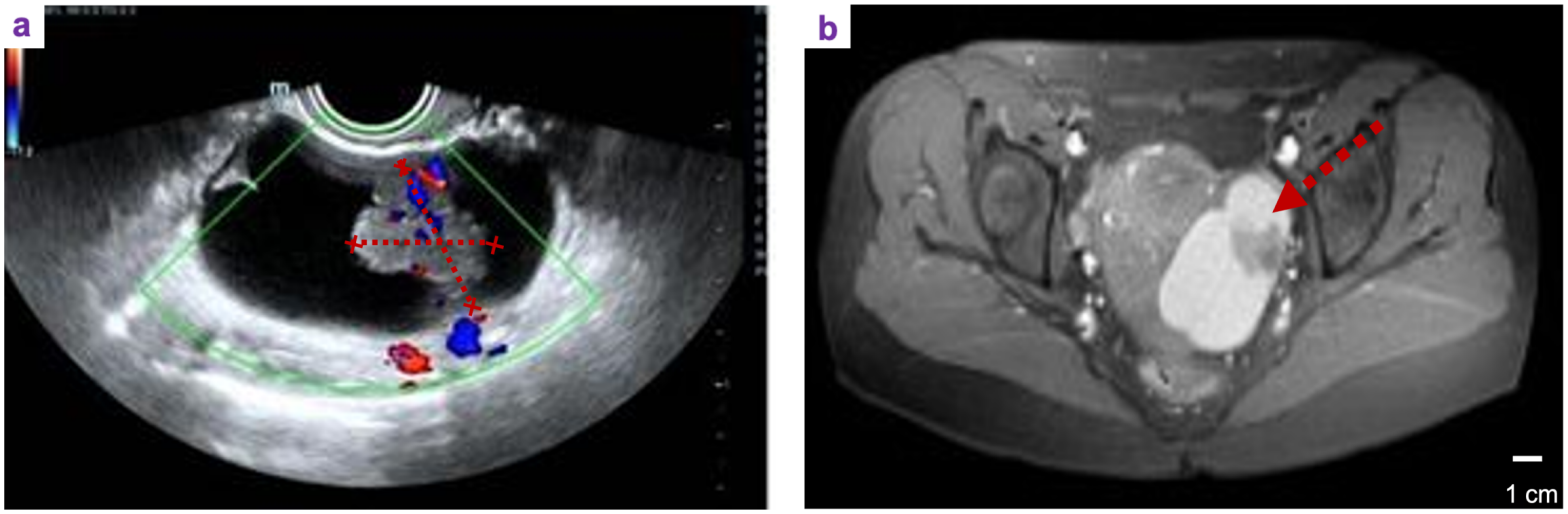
Preoperative imaging findings. (a) Transvaginal ultrasonography demonstrating a mixed cystic–solid mass in the left adnexal region with internal septations and increased vascularity on color Doppler imaging (arrow). (b) Axial pelvic magnetic resonance imaging (MRI) showing a cystic–solid lesion in the left adnexa with enhancing solid components (arrow).
